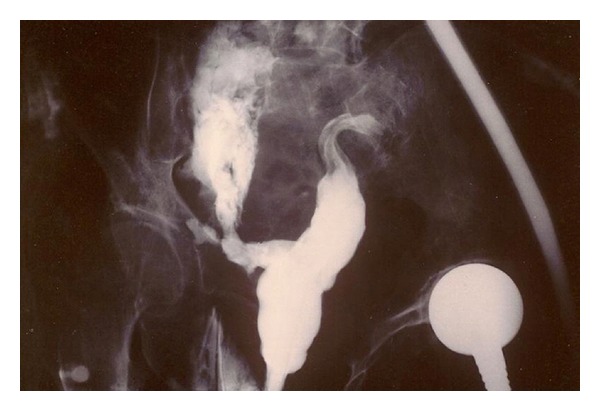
Plain anteroposterior radiograph showing the pelvis 5 minutes after per rectum administration of gastrografin. A rectal perforation allows gastrografin to escape into the pelvic cavity.
